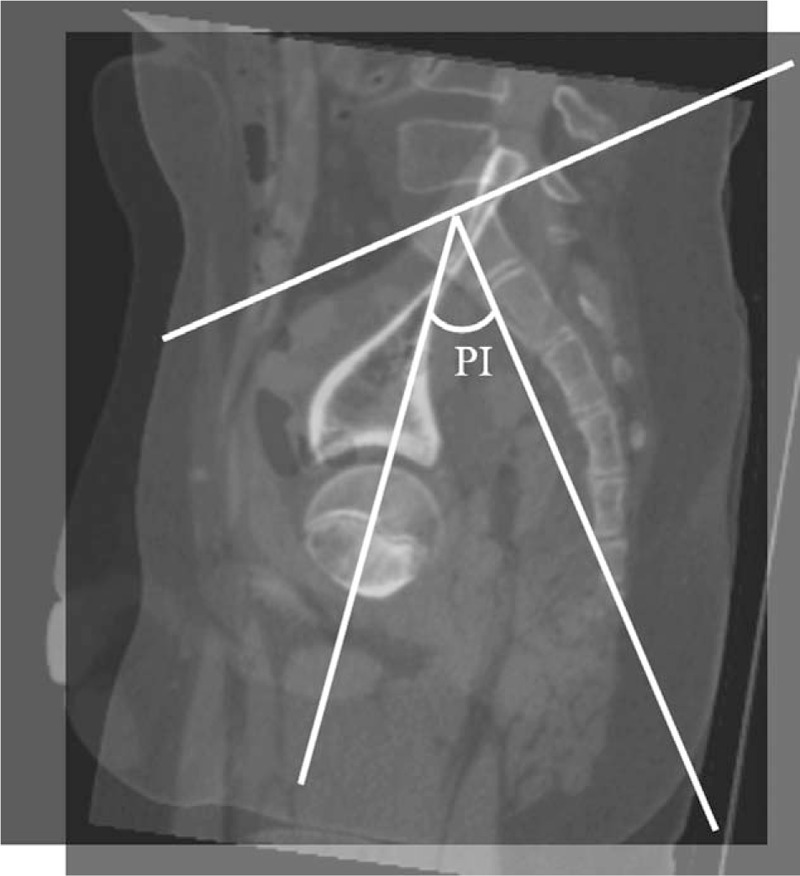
Pelvic incidence (PI) measurement as shown from the sagittal planes of computed tomography.
